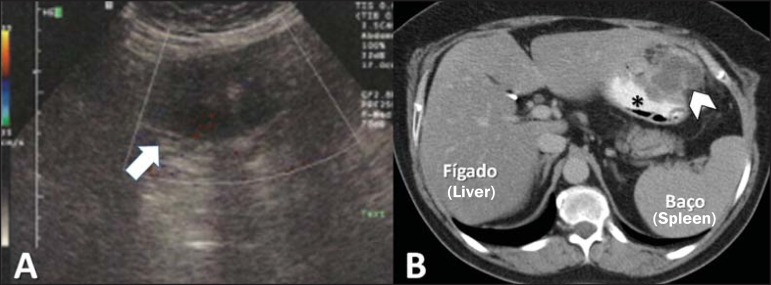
A: Ultrasound showing a septated cystic mass in the left
